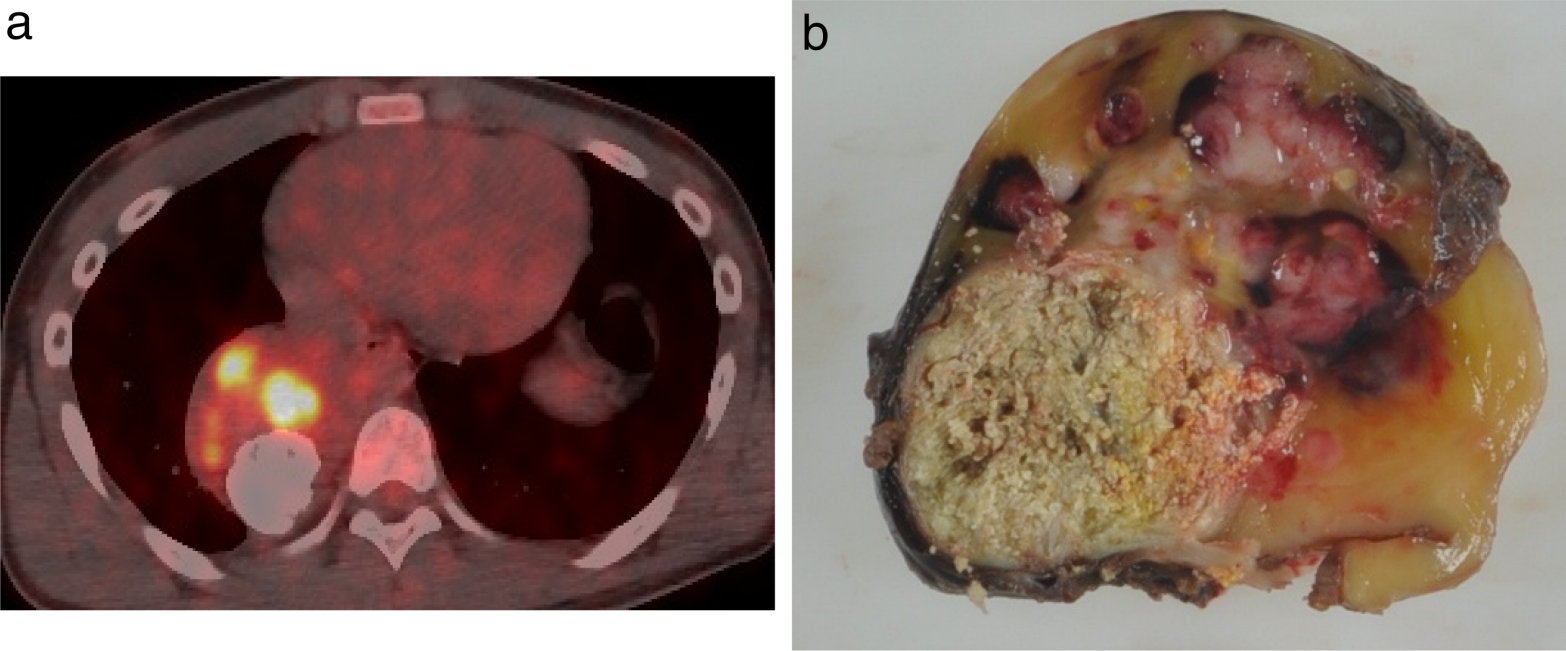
(a) Positron emission tomography with fluorodeoxyglucose‐computed tomography (FDG‐PET/CT) showed areas of increased FDG uptake within the tumor. (b) Macroscopic section of the resected tumor. The mass measured 13.5 × 7.5 × 6 cm and was variegated tan‐yellow in appearance with aggregated calcification and focal necrosis. Raised, dome‐shaped, white‐pink irregular nodules were seen inside the capsule.
